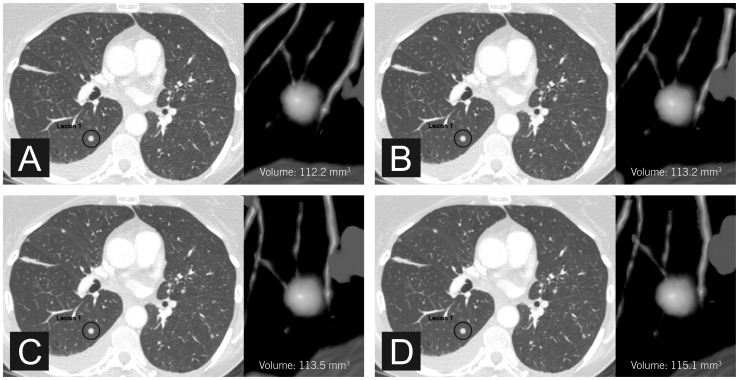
Axial CT images of a small pulmonary nodule. Measured volumes were 112.2 mm^3^ (A, FBP), 113.2 mm^3^ (B, iDose^4^ level 2), 113.5 mm^3^ (C, iDose^4^ level 4), and 115.1 mm^3^ (D, iDose^4^ level 6).
